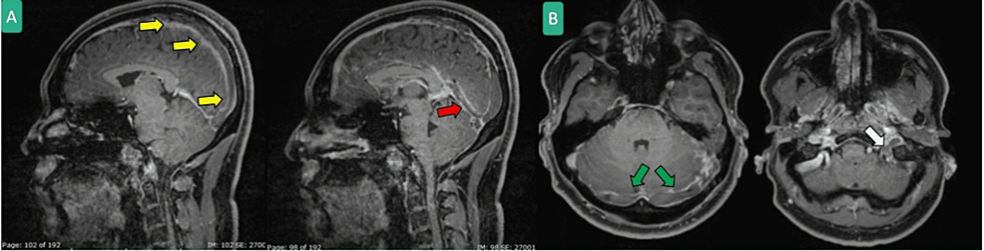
Head MRI with contrast MPRAGE sequence sagittal (A) and axial (B) showing extensive cerebral venous thrombosis in superior sagittal (yellow arrows), straight (red arrow), bilateral transverse (green arrow) and sigmoid/jugular sinuses (white arrows).
